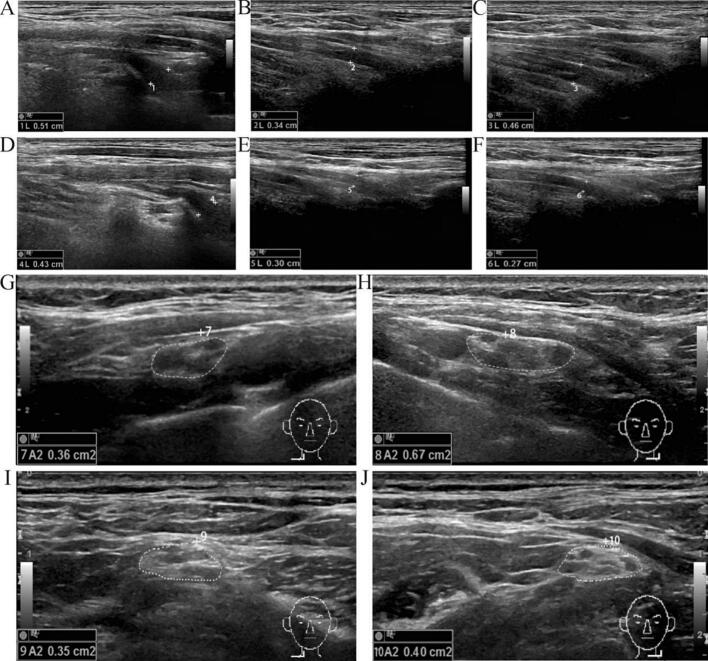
Dynamic ultrasonographic evolution of brachial plexus nerve roots following carbon monoxide poisoning. (A-C) Acute phase (Day 19) longitudinal ultrasonographic images of left C5, C6, and C7 nerve roots: Demonstrate marked nerve root swelling and thickening with reduced echogenicity and loss of normal fascicular architecture. Measured nerve root thicknesses are 5.1 mm (C5), 3.4 mm (C6), and 4.6 mm (C7), respectively. (D-F) Acute phase (Day 19) longitudinal ultrasonographic images of right C5, C6, and C7 nerve roots: Serving as the unaffected contralateral control, the right-sided nerve roots display normal echogenicity and preserved fascicular structure, with measured thicknesses of 4.3 mm (C5), 3.0 mm (C6), and 2.7 mm (C7), respectively. Bilateral comparison reveals obvious swelling and thickening of the left-sided nerve roots. (G-H) Acute phase (Day 19) axial ultrasonographic comparison: The left brachial plexus nerve roots (G) show significant fusiform swelling with blurred fascicular architecture and heterogeneous echogenicity. The C5-C7 nerve root complex demonstrates a cross-sectional area of approximately 67 mm^2^. The right brachial plexus nerve roots (H) maintain normal morphology with a cross-sectional area of approximately 36 mm^2^. The left-to-right cross-sectional area ratio of 1.86 indicates marked asymmetric swelling. (I-J) Recovery phase (Day 56) axial ultrasonographic comparison: The left brachial plexus nerve roots (I) show partial structural recovery with gradually improving fascicular definition and enhanced echo texture, though residual swelling persists with a cross-sectional area of approximately 42 mm^2^. The right brachial plexus nerve roots (J) maintain normal morphology with a cross-sectional area of approximately 35 mm^2^. The left-to-right cross-sectional area ratio decreased to 1.20, demonstrating significant recovery trends. Technical specifications: Ultrasonographic examinations were performed using a 12–15 MHz high-frequency linear array transducer with the patient positioned supine and the neck in mild extension. Measurements were obtained under standardized conditions with appropriate probe pressure and gain settings.
